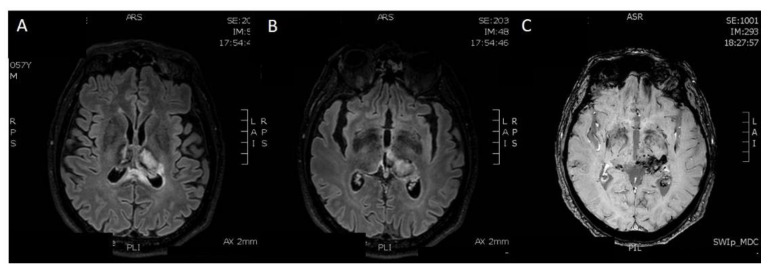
Five months later, he underwent a brain MRI exam. (A,B) Axial FLAIR-weighted sequences show reduction in the diameter of haemorrhagic focus and reduction in perilesional oedema in both thalami. (C) In the Susceptibility-Weighted Images, old hematic deposits can be found in both thalami, particularly on the left. Therefore, the exam showed a tendency to encephalomalacic transformation of the ischemic regions and improved sinus rectum patency, with some wall irregularities. The patient continued treatment with Enoxaparin.
